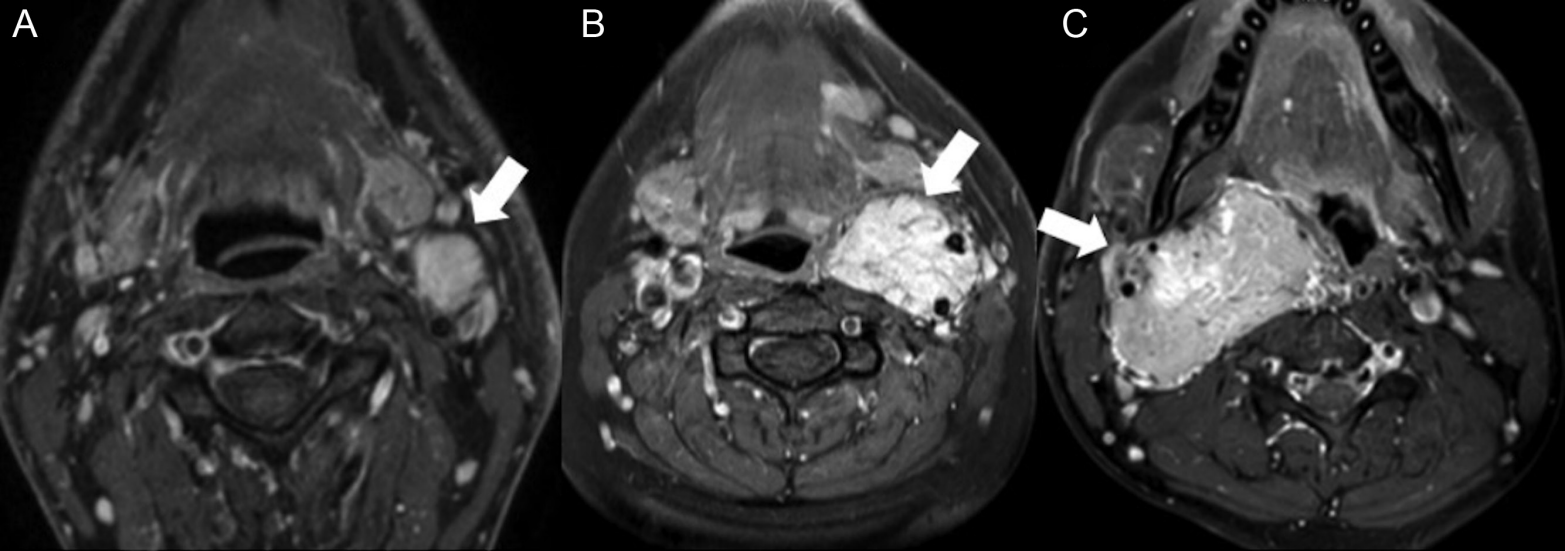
Axial post-contrast MRI findings for Shamblin class I CBT (A), class II CBT (B), and class III CBT (C).
